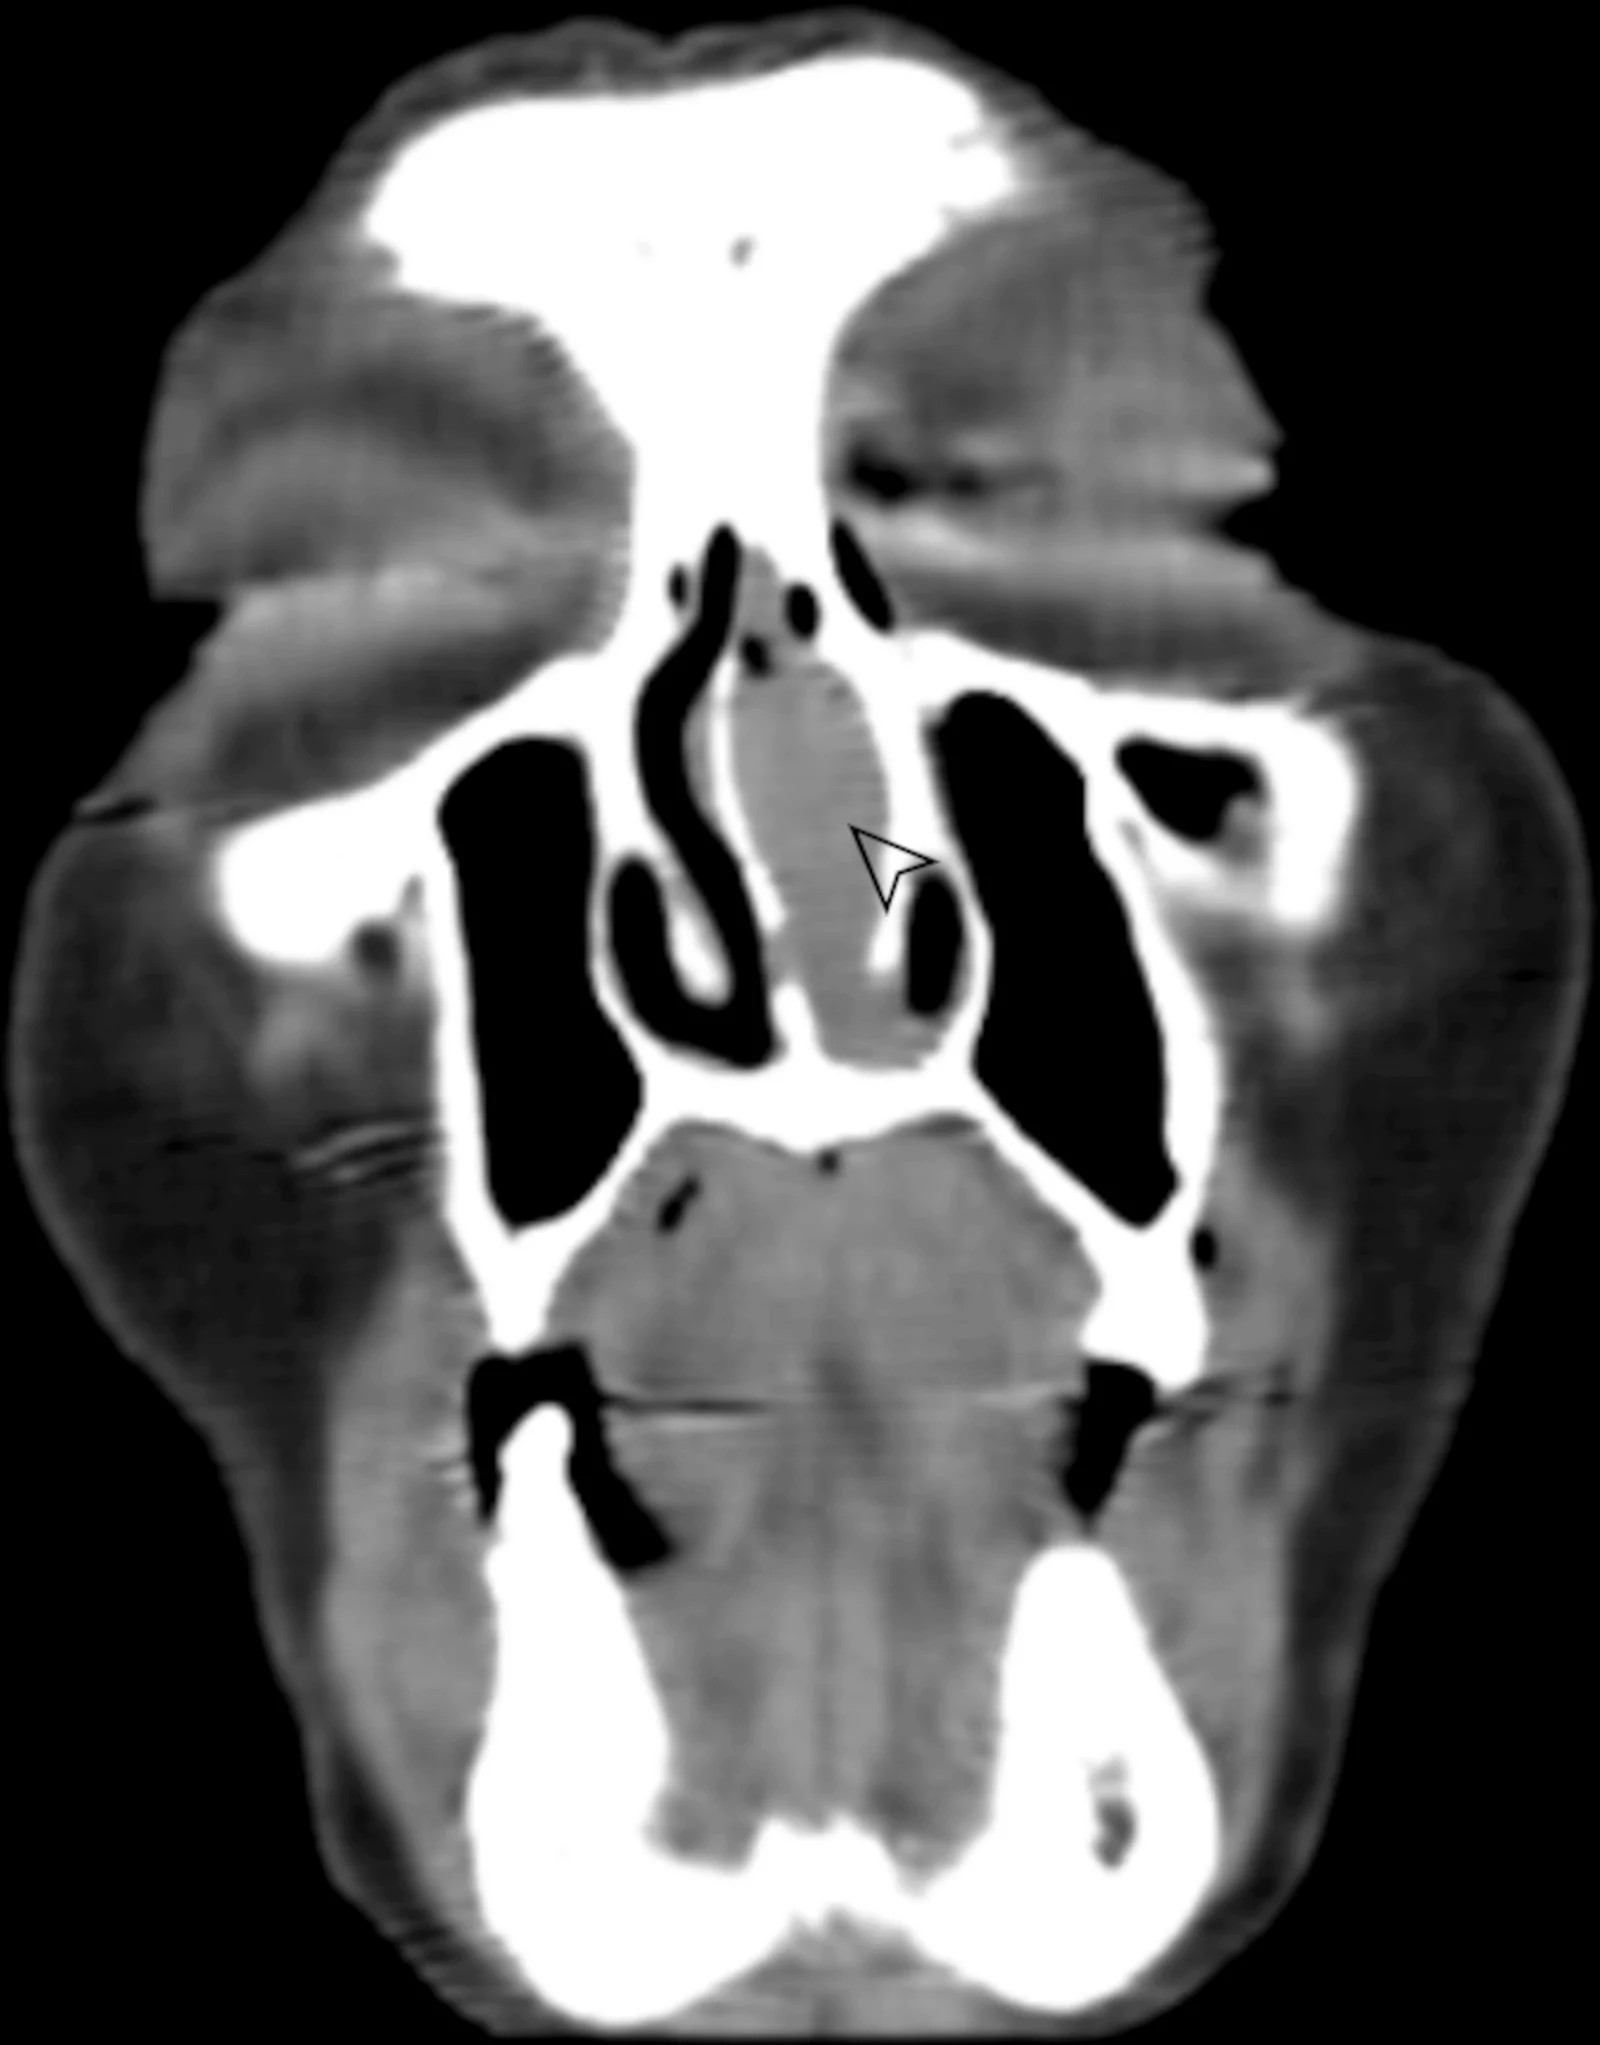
Computed tomography of the paranasal sinuses and nasal cavities (coronal view) Coronal CT image of the paranasal sinuses and nasal cavities showing a hypodense solid mass (39 Hounsfield units (HU) on basal imaging and 44 HU after contrast administration) arising from the left inferior turbinate at the superolateral aspect of the nasal cavity and projecting into the nasal cavity (arrowhead). No evidence of adjacent bone erosion or invasion of the paranasal sinuses or skull base was observed.
